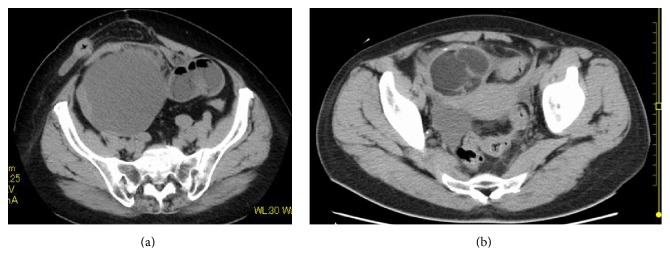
(a) Abdominal plain computed tomography (current). A huge mass measuring 21 cm in longest diameter can be seen in the right cranial portion of the uterine body. The mass is partially solid, suggesting malignancy. (b) Abdominal plain computed tomography (image obtained 10 years prior to the current admission). A mature cystic teratoma measuring 6 cm in diameter is observed on the right side. There are no findings suggestive of malignancy, such as a mural nodule or a solid component.
